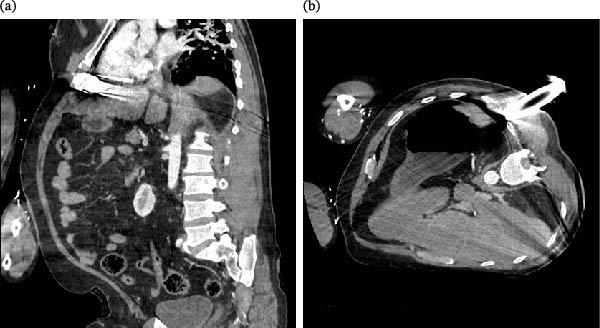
(a and b) Trauma computed tomography (CT) scan excluded cardiac, pericardial, mediastinal, tracheal, esophageal, or parenchymal damage amenable to treatment. The diaphragm is best assessed intraoperatively. The image shows that the metallic tube entered the chest through the 10th posterior left intercostal space, and is lying on the left hemidiaphragm adjacent to the heart and lung.
